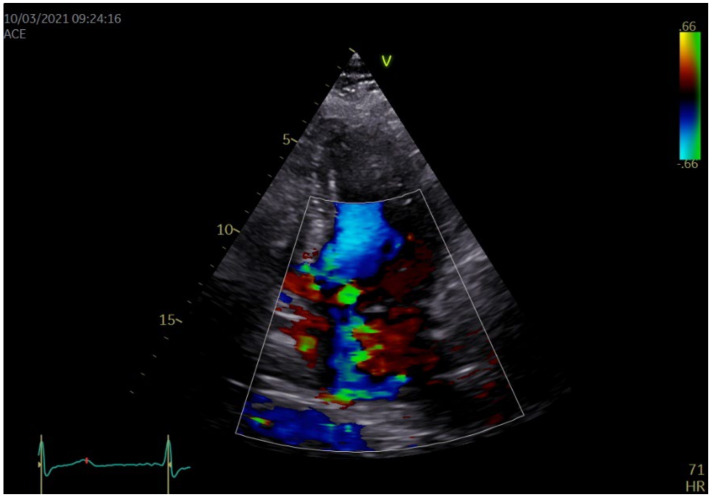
Transthoracic bidimensional echocardiography, four chamber view with color Doppler on the mitral valve: severe mitral valve regurgitation, with a central slightly posterolateral deviated jet. The regurgitant jet enters the pulmonary veins.
